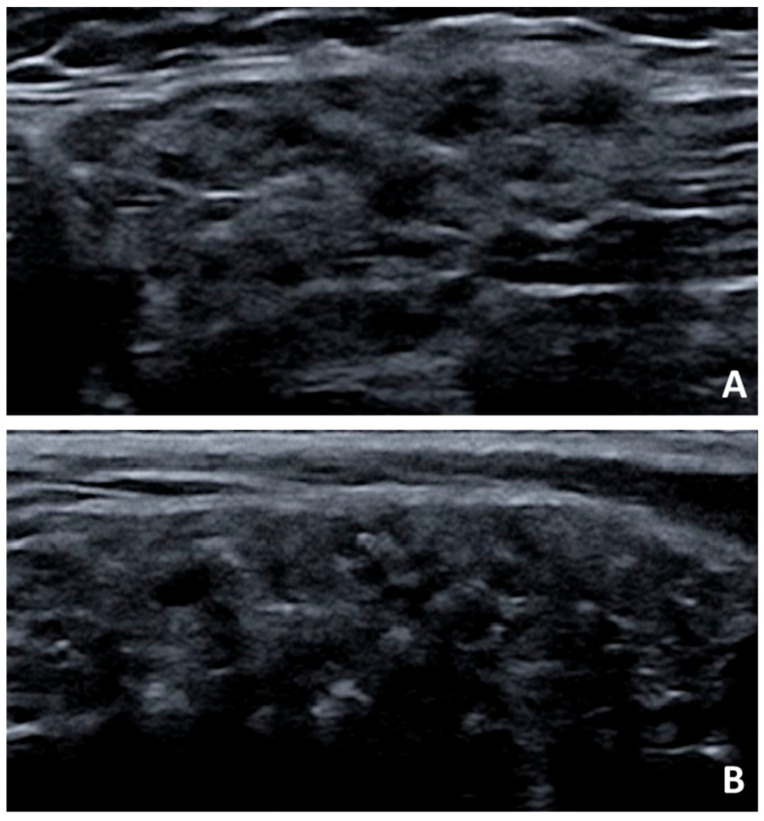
Salivary gland ultrasonography findings in pSS. Presence of hypoechogenic areas and hyperechogenic reflections in the (A) submandibular gland and (B) parotid gland of a pSS patient.
